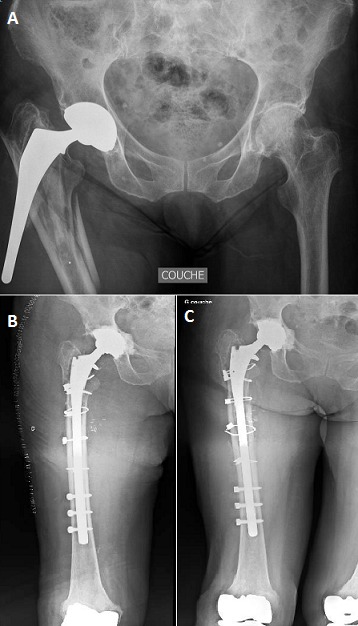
A): radiographie du bassin montrant le descellement important de la tige suite à la fracture de fémur ; B): contrôle post opératoire immédiat de la prothèse de reprise (tige fémorale et cotyle) avec la bonne réduction de la fracture fémur :C) : contrôle après 6 mois montrant la consolidation et la bonne prise de la prothèse
